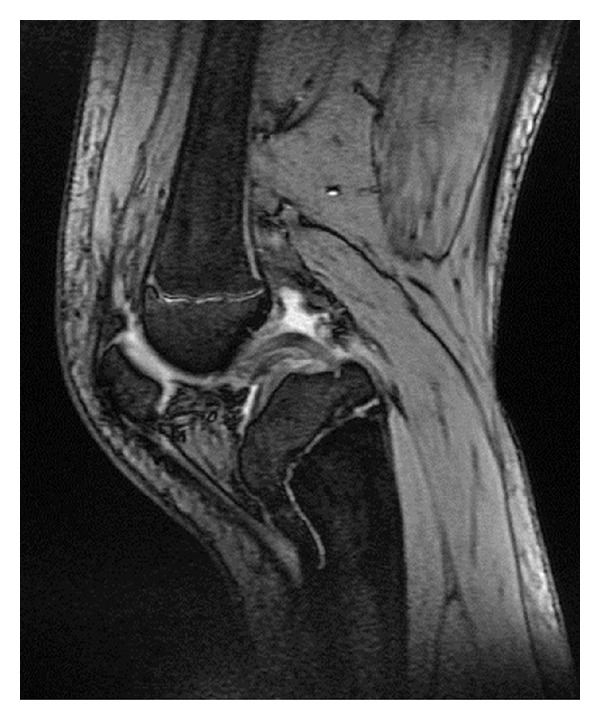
MRI of the left knee: narrowing of the proximal tibial growth plate, arrest of the tibial tubercle, thickening and shortening of the extensor mechanism, relaxation of the posterior cruciate ligament, and posterior subluxation of the joint.
